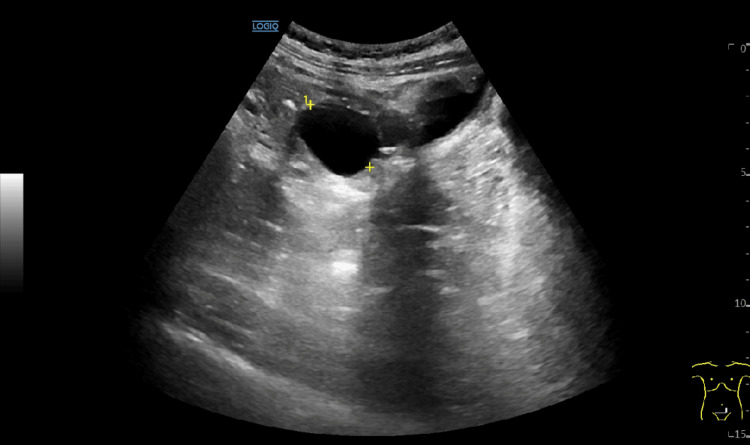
Gynecological ultrasound. Gynecological ultrasound revealed two adnexal cysts on the left measuring 2.7 and 3.7 cm, of simple appearance, probably functional.
